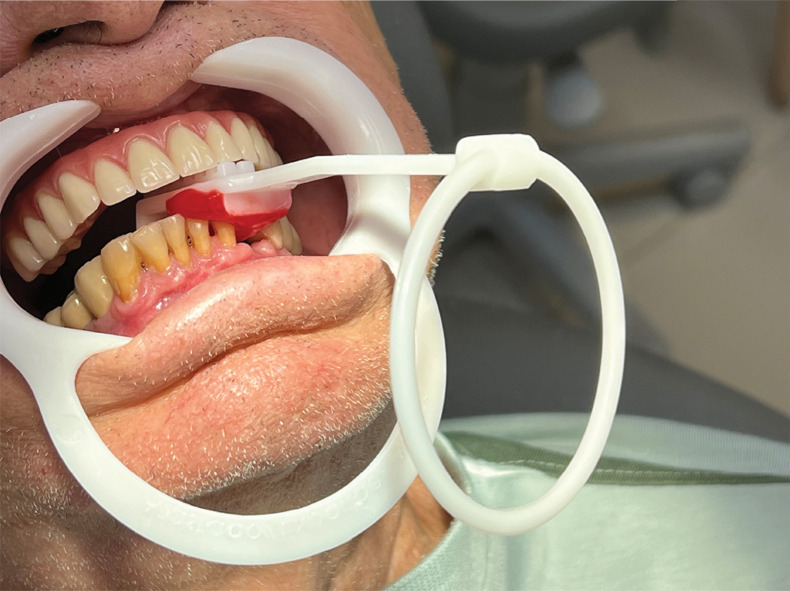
Device for standardizing radiographs.
